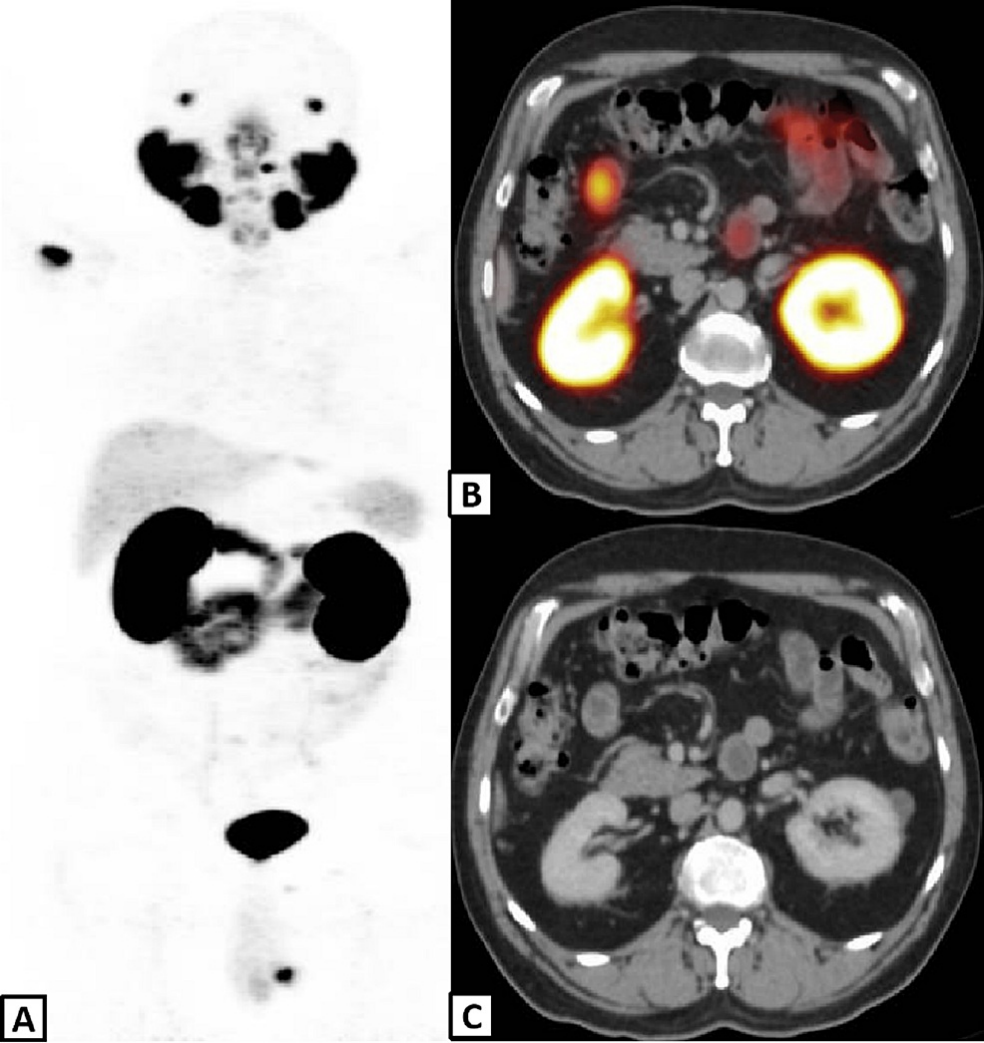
PSMA PET-CT scan Maximum intensity projection (A), fused axial PET-CT (B) and axial CT (C) slices show focal osseous metastasis in right humerus and exophytic mass in left kidney.
